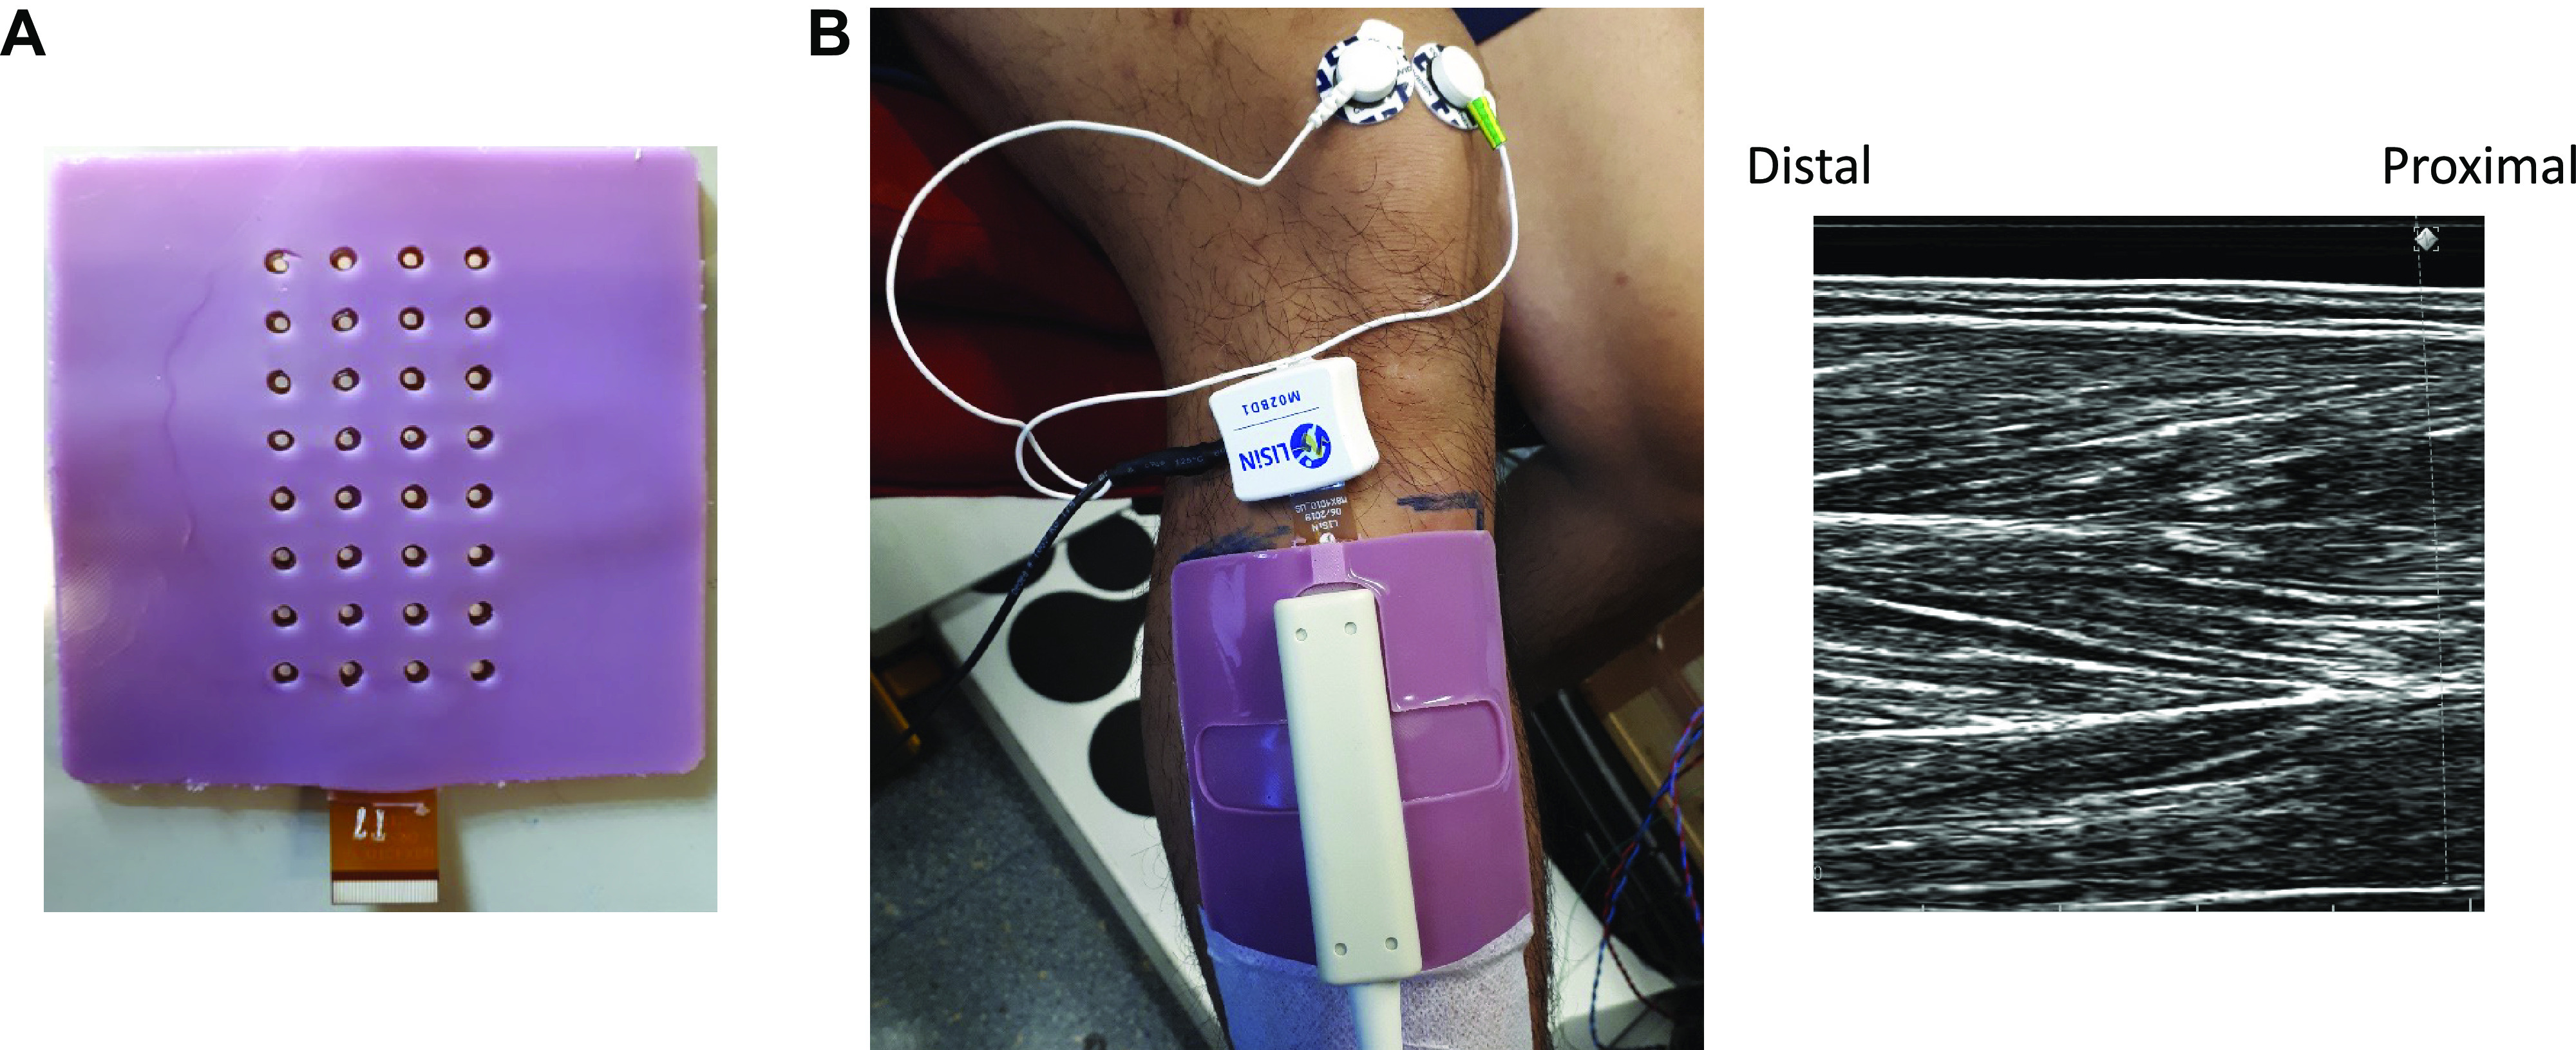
High-density surface electromyography (HDEMG) ultrasound-transparent electrodes. *A*: 8 × 4, 32-channel (10-mm interelectrode distance) electrode grid. *B*: HDEMG electrode grid with 32-channel HDEMG amplifier (connected on top of the electrode) and flat ultrasound probe can be seen on the left. Ultrasound image of proximal tibialis anterior muscle can be seen on the right. Note the quality of the image with accurate visualization of fascicles and, superficial, intermediate, and deep aponeuroses (*n* = 1, male participant).
